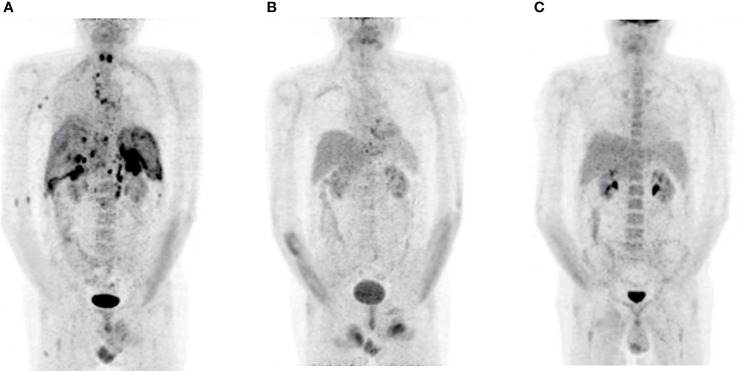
FDG PET imaging patient 1. (A) Relapse 6 months after the therapy with CAR T-cells (prior to Pola-BR). (B) Complete metabolic remission after three courses of Pola-BR. (C) Confirmed complete metabolic remission 12 weeks after alloHSCT.
